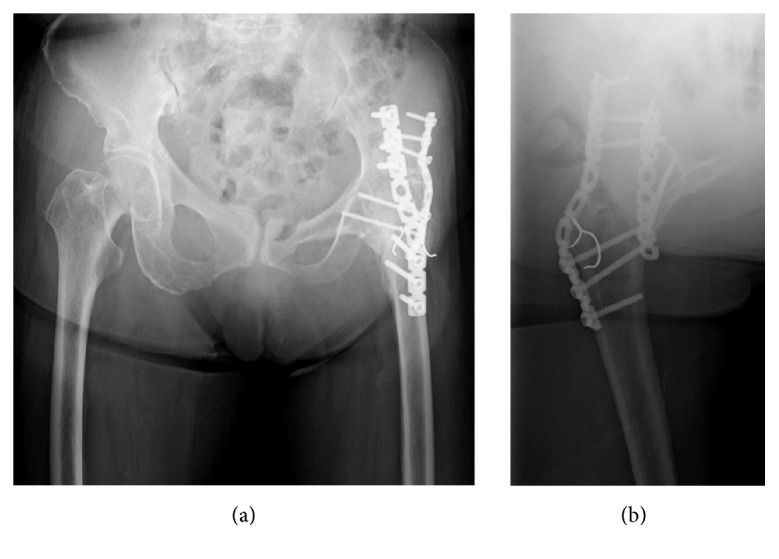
Follow-up radiographs at 10 months after surgery. (a) AP view and (b) lateral view. Bone union of fracture site was revealed with no displacement and no implant complication.
